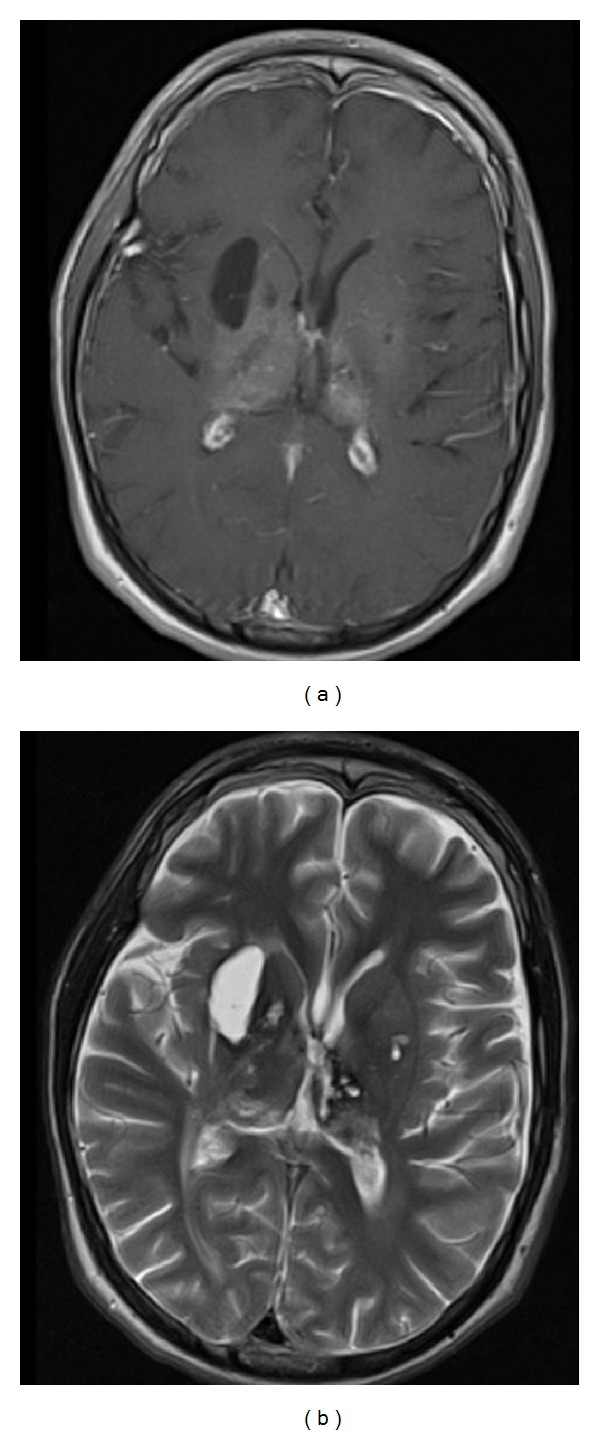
MRI 10 months after radiation treatment. (a) T1-weighted, axial, gadolinium-enhanced image at level of foramen of Monro. (b) T2-weighted, axial image at same level.
